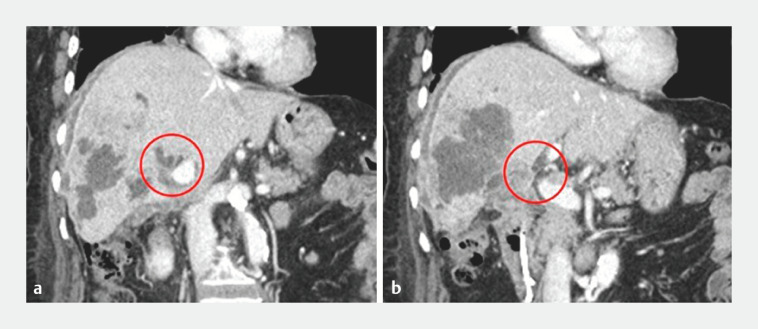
Enhanced computed tomography reveals bilateral intra-bile duct dilation.
